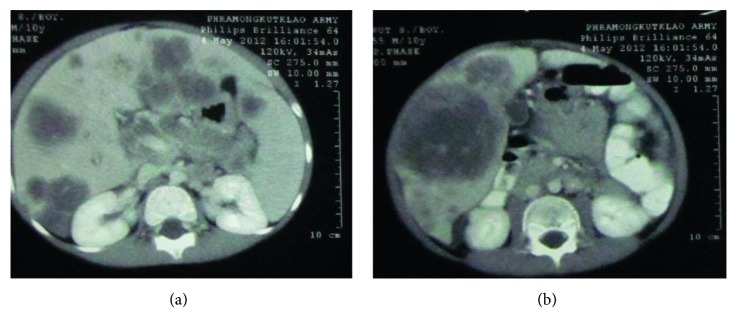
CT chest and whole abdomen (axial view). (a) Multiple masses at both lobes of the liver, the spleen, and the pancreatic body and tail. (b) The largest hepatic mass of 8 × 7.5 × 11.6 cm in diameter.
